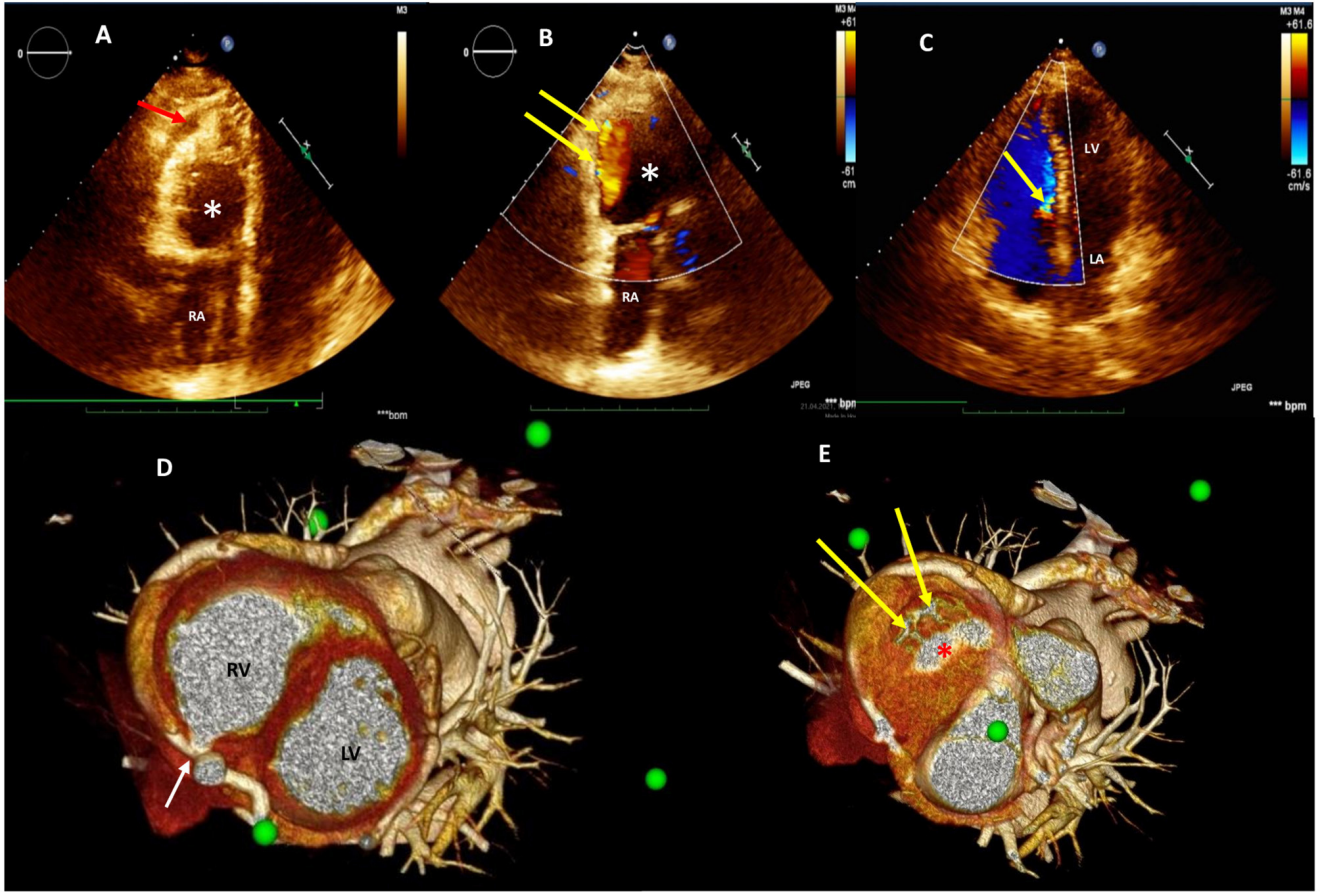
Two-dimensional (2D) transthoracic echocardiogram (TTE) color Doppler showing significant deformation and compression of the right ventricular cavity (red arrow) due to the presence of additional thin-wall round-shaped chamber (white asterisk) (A); two coronary-right ventricle fistulas draining into the additional round-shaped chamber (B); dislocation of the tricuspid valve structures toward the ventricular septum with marked tricuspid flow reduction (yellow arrow) (C); pre-operative assessment by three-dimensional multidetector CT angiography (3D-MDCTA) volume-rendering reconstruction showing entry point (white arrow) (D) and two exit sites (yellow arrows) draining into a round-shaped chamber (red asterisk) (E) within the right ventricle. 3D-MDCTA, three-dimensional multidetector computed tomography angiography; RA, right atrium; RV, right ventricle; LA, left atrium; LV, left ventricle.
